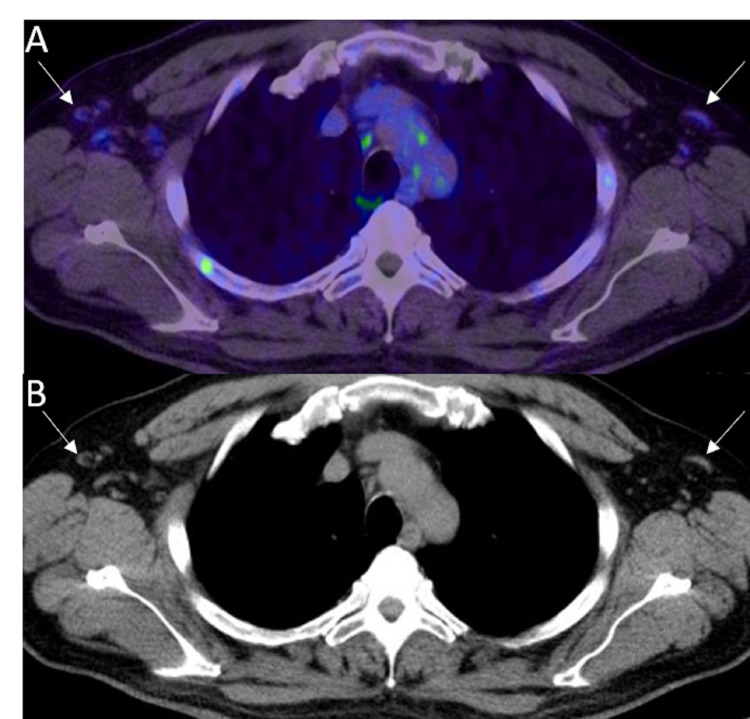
PSMA-RADS-1B A: Axial 68Ga PSMA PET/CT through the chest shows mild uptake in benign small axillary lymph nodes with fatty hila (white arrows). B: Axial CT through the chest in the same patient shows correlate benign small axillary lymph nodes with fatty hila (white arrows).
